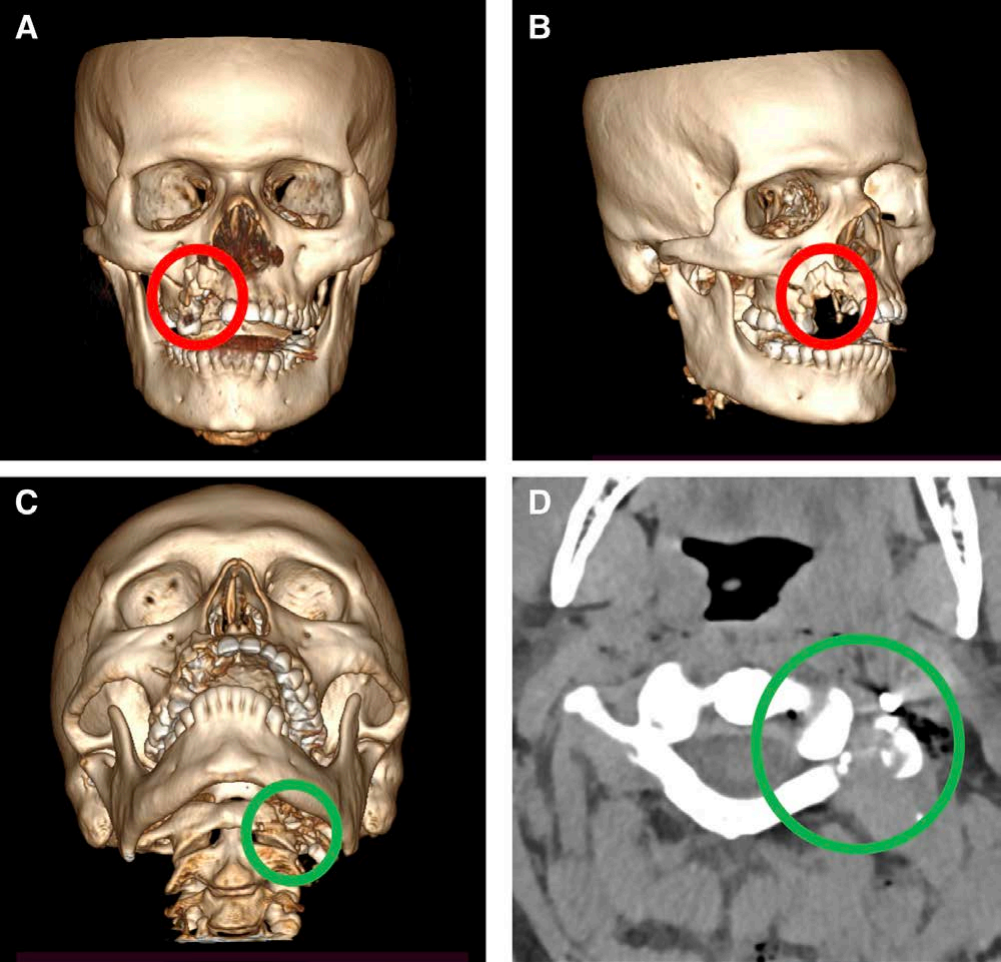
Preoperative multi-detector computed tomography. (A and B) Bone reconstruction in 3 dimensions showed a fracture of the body, lateral and inferior wall of the maxillary sinus on the right - red circle. (C) Bone reconstruction in 3 dimensions showed complex fracture was observed in the anterior arch, lateral mass, and in the left transverse process of the C1 cervical vertebra. (D) Coronal section of C1 cervical vertebra showing fracture with bone fragments and lodged bullet with light enhancement.
